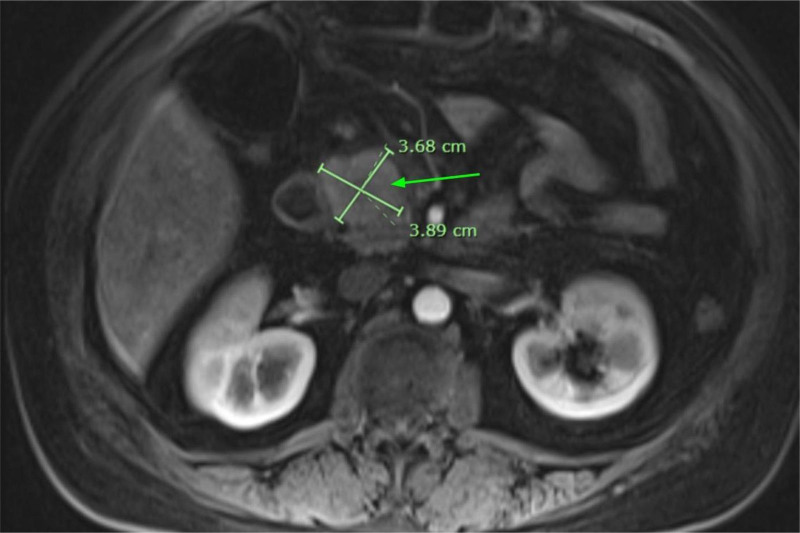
Magnetic resonance imaging abdomen with and without contrast with magnetic resonance cholangiopancreatography. MRI of the abdomen revealed a 3.9 cm mass on the head of the pancreas (green arrow) concerning for adenocarcinoma versus focal inflammatory change. MRI = magnetic resonance imaging.
